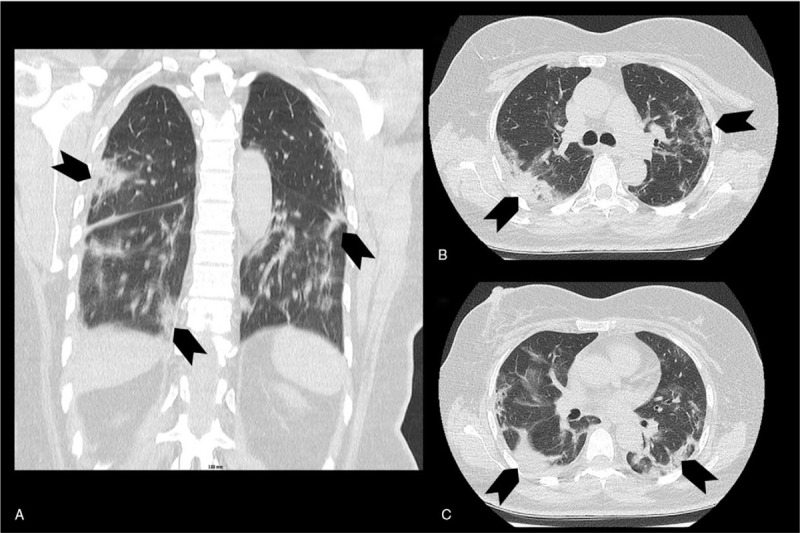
Patient (case 1) chest CT scan. Chest CT scan (A: frontal view, B and C: axial views) showing typical pneumonia due to SARS-Cov-2 infection with ground glass opacities (arrows).
